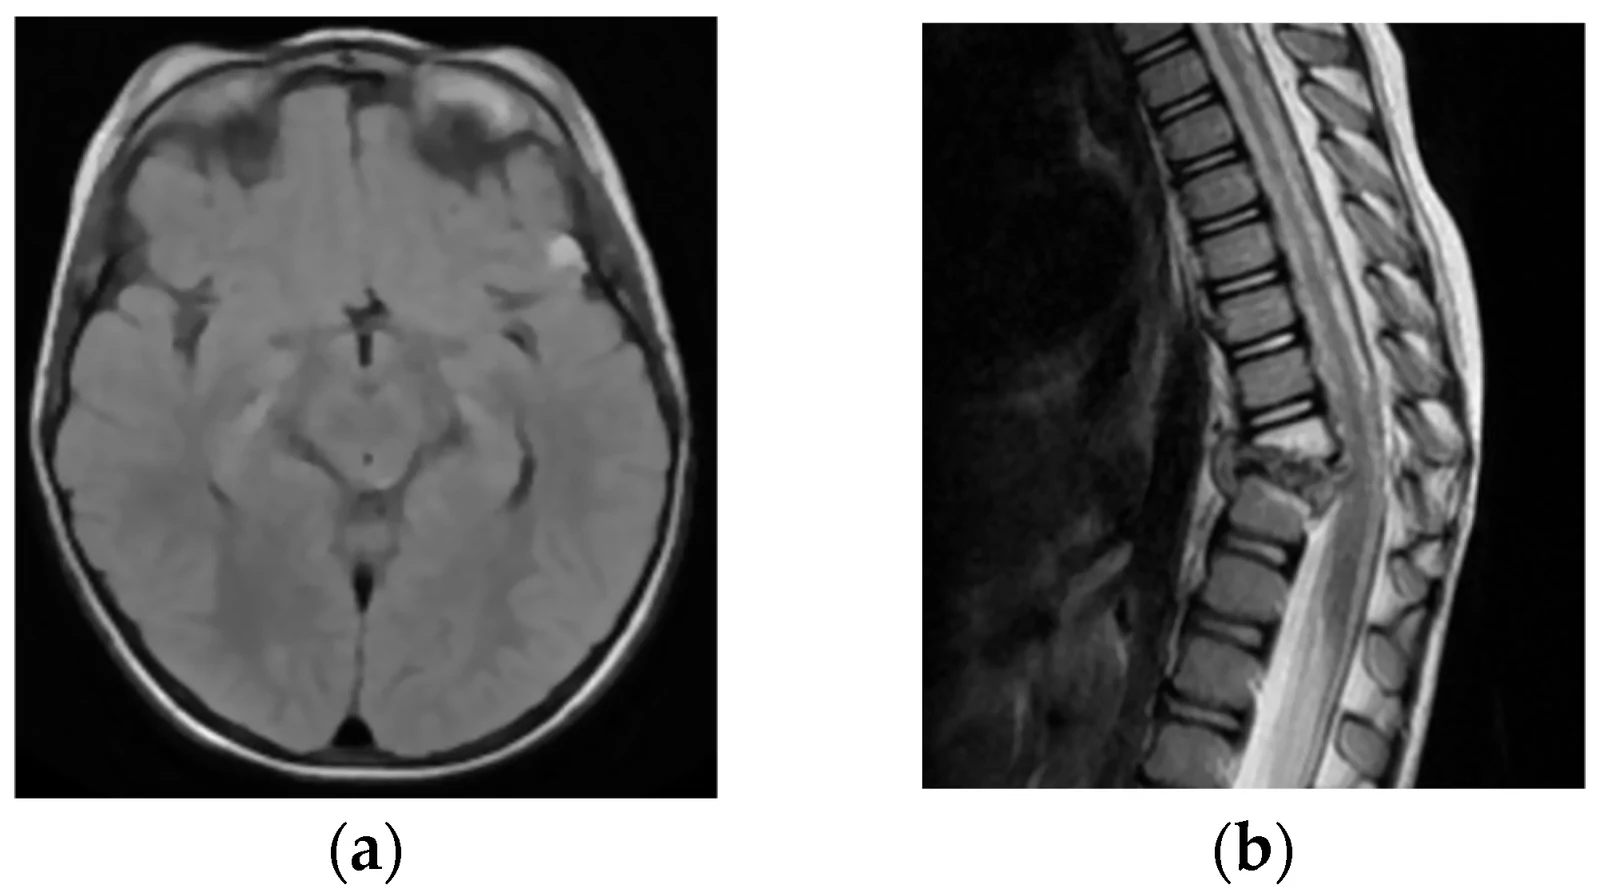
One-month follow-up MRI of the brain and spine: (a) reduction in the size of the left frontal tuberculoma; (b) marked decrease in the paravertebral abscess.
